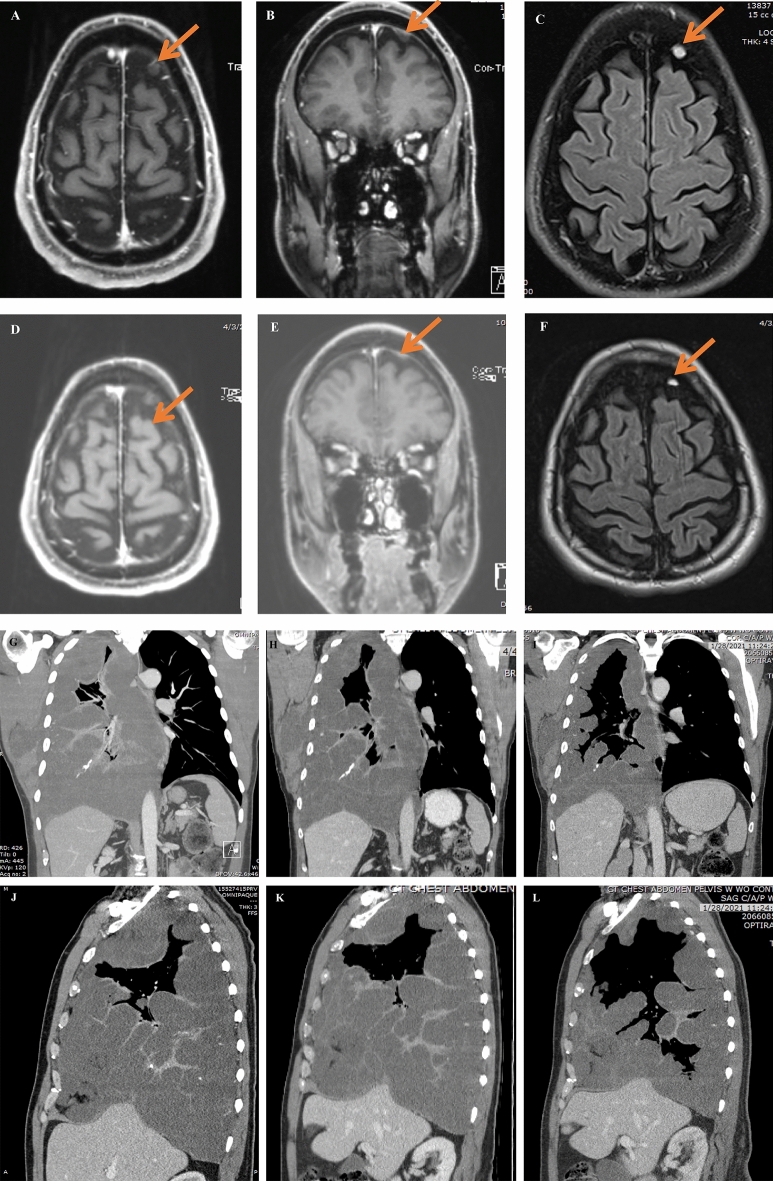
Case 2 MRI Brain and MRI Chest. MRI Brain: Post-contrast MRI of patient with metastatic chordoma in dura of brain (A-axial, B-coronal) and on T2/FLAIR (C) showing the lesion before starting pemetrexed 900 mg/m^2^ and 6 weeks post-treatment in brain (D-axial, E-coronal) and on T2/FLAIR (F) showing response. CT Chest: Post-contrast Chest CT of patient with metastatic chordoma to lung and soft tissue of axilla (G-coronal, J-sagittal) showing the large lesion filling most of right lung before starting pemetrexed 900 mg/m^2^, 6 weeks post-treatment (H-coronal, K-sagittal) showing shrinkage of tumor, and 2 years later on pemetrexed showing continued shrinkage of tumor and expansion of air ways in right lung and reduced mass effect and shift of mediastinal contents (I-coronal, L-sagittal).
